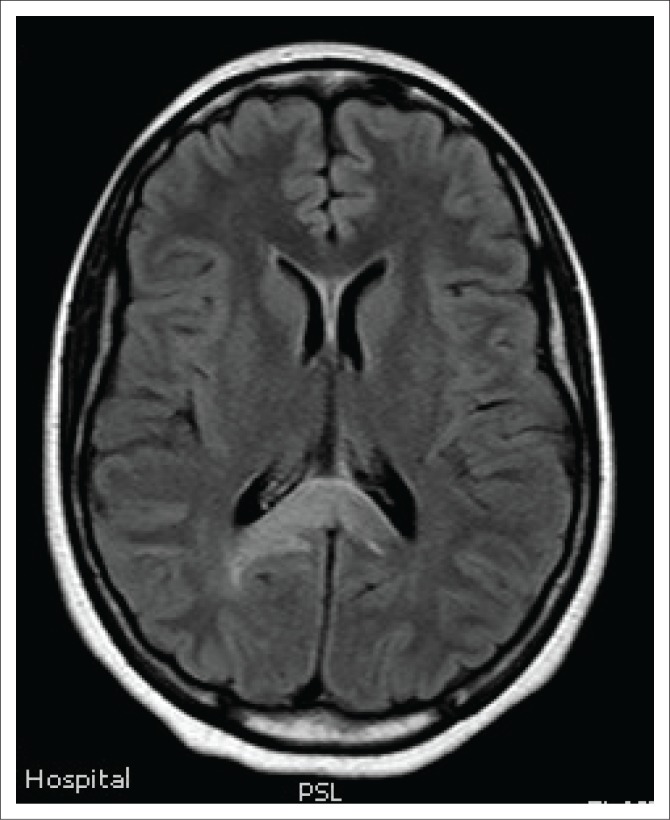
Axial fluid-attenuated inversion recovery magnetic resonance image demonstrating hyperintensity in the splenium of corpus callosum in a ‘bridging arch pattern’.
